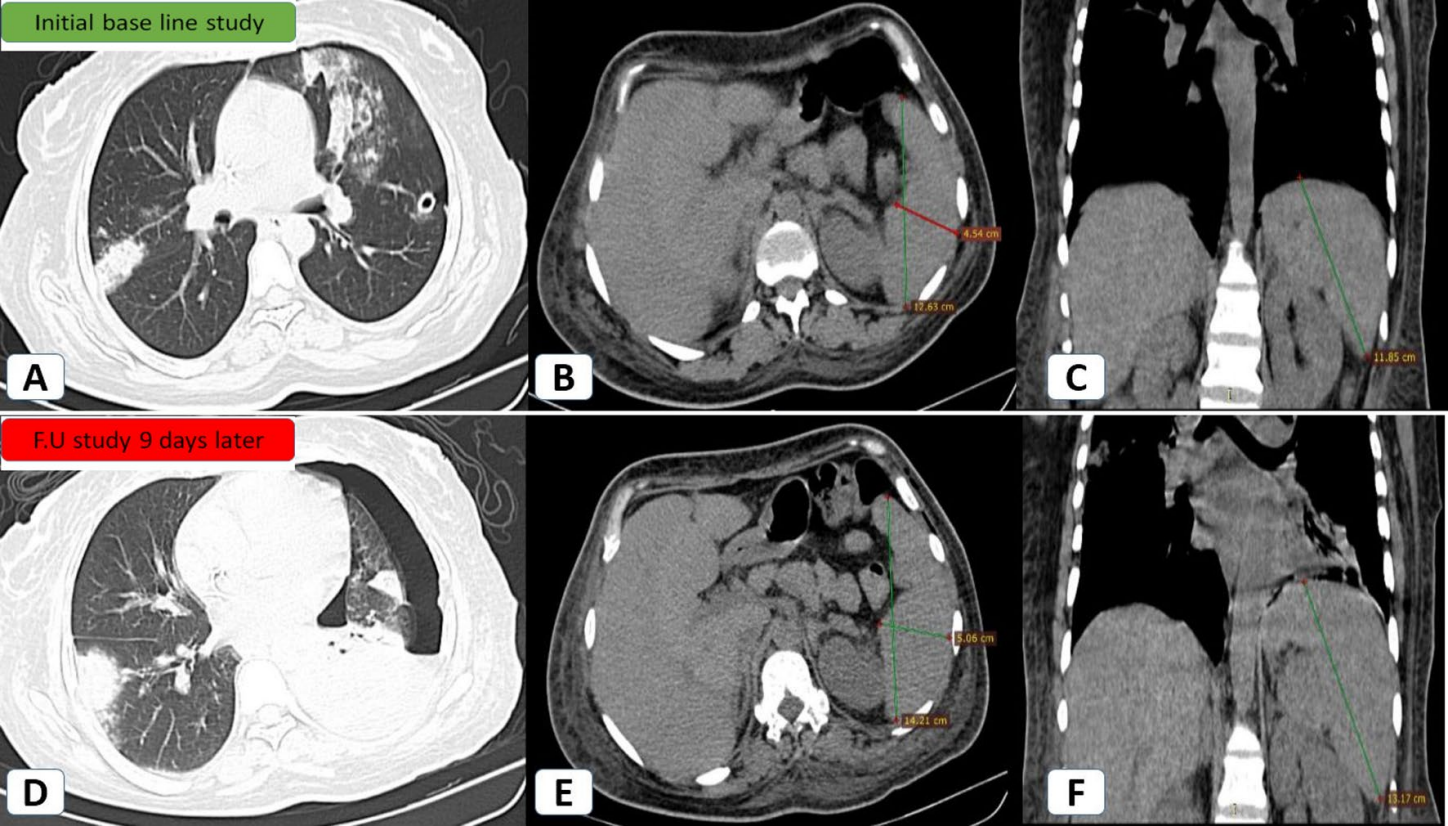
Progressive splenomegaly in a 43-year-old female patient with severe COVID-19 infection and elevated Interleukin-6 (320 pg/ml), consistent with initiation of cytokine storm: A–C Initial baseline study: A Axial HRCT (lung window) showing right posterior segmental upper lobar sub-pleural ground glass patch and left upper lobar confluent ground opacities. Minimal left-sided pneumothorax was noted with an inserted inter-costal tube. B Axial CT (mediastinal window) for the maximum anteroposterior and side-to-side splenic dimensions (12.6 cm and 4.5 cm). C Coronal CT (mediastinal window) for the maximum splenic craniocaudal dimension (11.8 cm). D–F Follow-up of the same patient nine days later: D Axial HRCT (lung window) showing left-sided progressing hydro-pneumothorax with denovo left lingular sub-pleural wedge-shaped patch. E Axial CT (mediastinal window) for the maximum anteroposterior and side-to-side dimensions (14.2 cm and 5 cm). F Coronal CT (mediastinal window) for the maximum craniocaudal dimension (13.1 cm)
